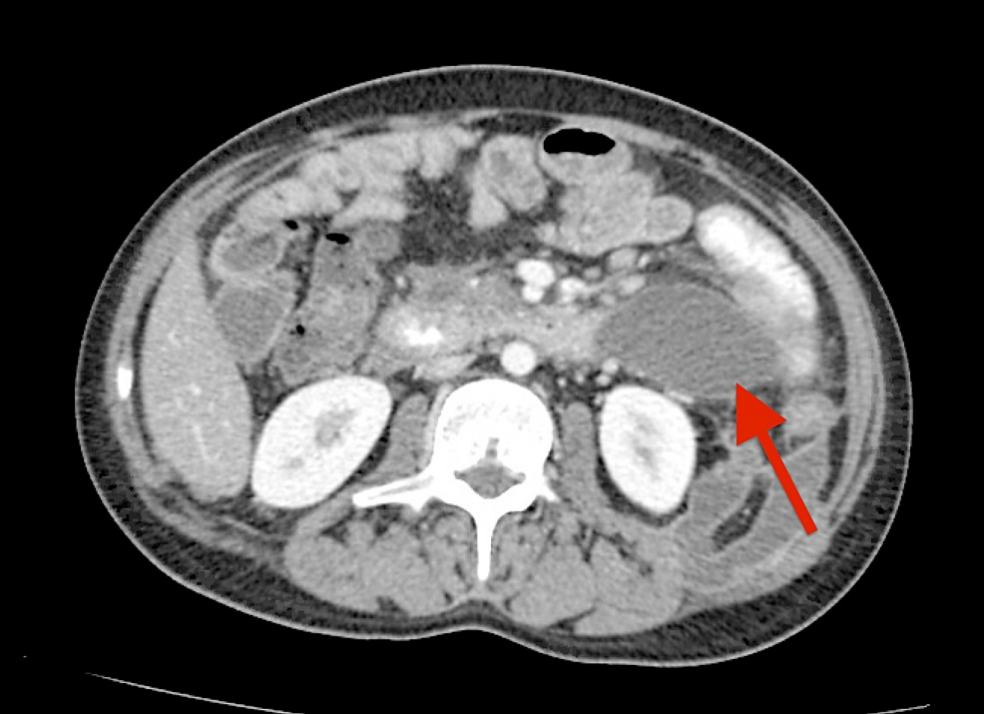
CT abdomen showing healing necrotizing pancreatitis complicated by the formation of a multiloculated pseudocyst (indicated by the red arrow)
